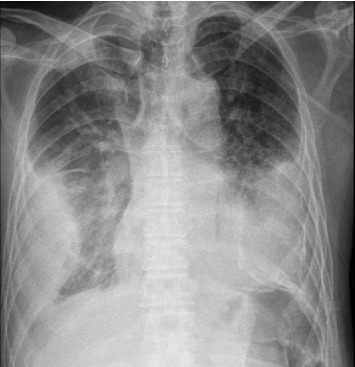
Chest x-ray at initial presentation demonstrating bilateral chronic pleural effusions.
